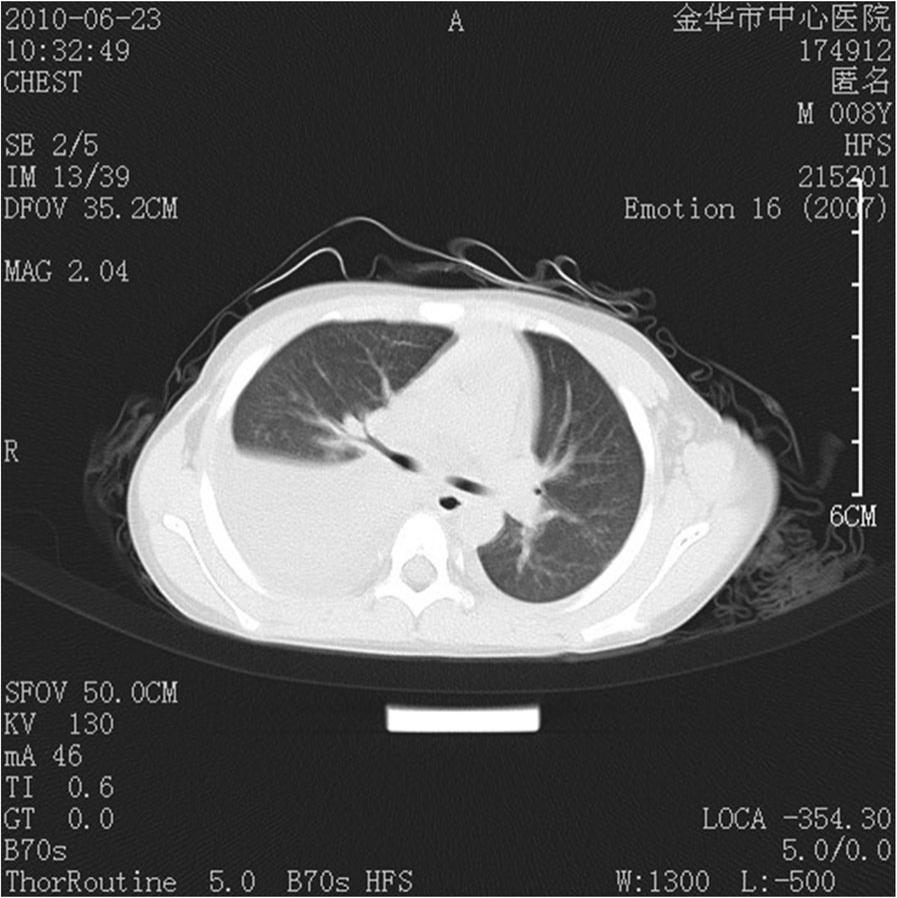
Chest CT: Large high-intensity lesions in his right lower lung lobe, the stenosis of the lower right bronchial lumen and right-sided pleural effusion at the initial presentation
